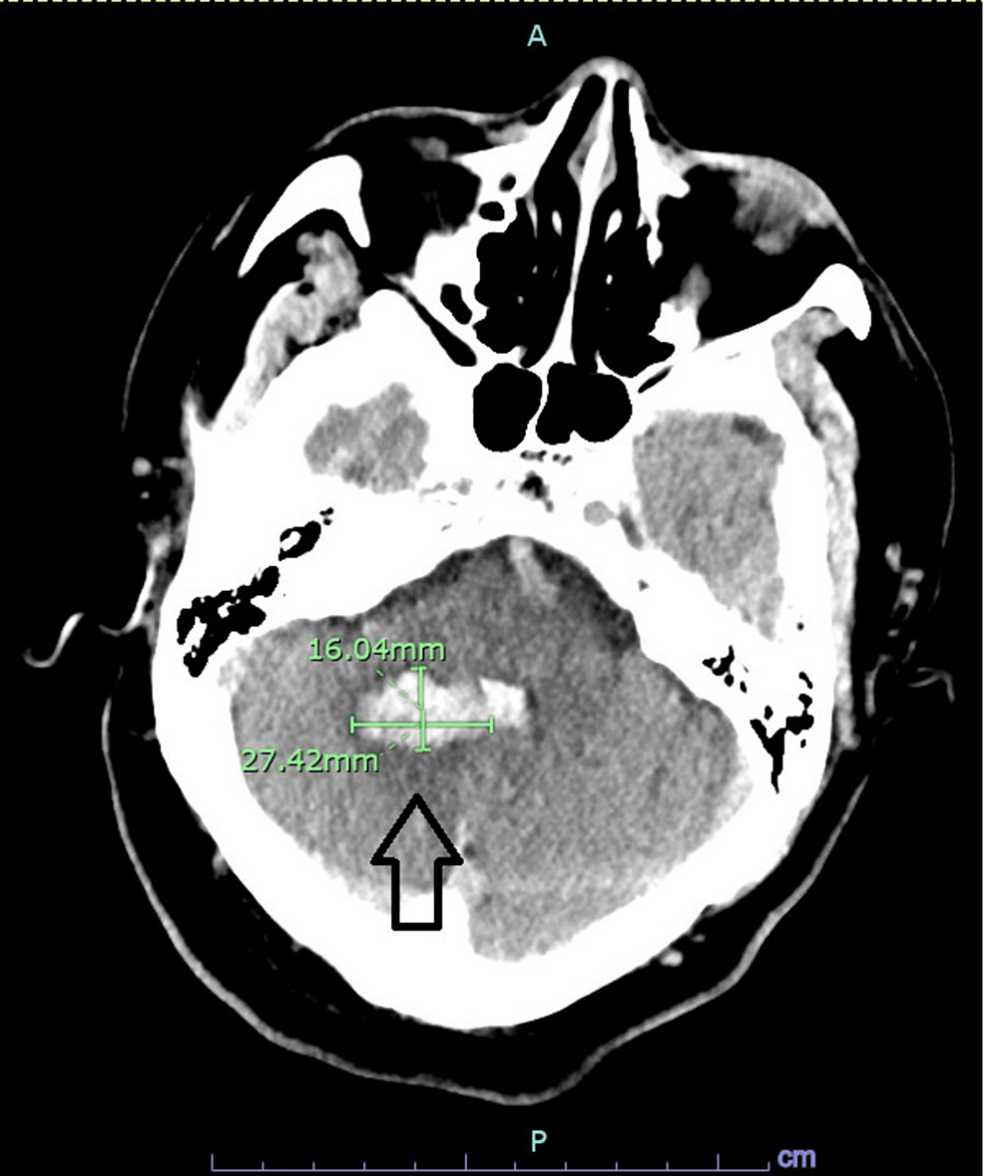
CT head Computed tomography (CT) head without contrast showing evidence of acute right-sided cerebellar hemorrhage affecting the superior cerebellar peduncle with extension to the fourth ventricle, left-sided displacement of the cerebral aqueduct and surrounding cerebral edema without hydrocephalus (arrow)
